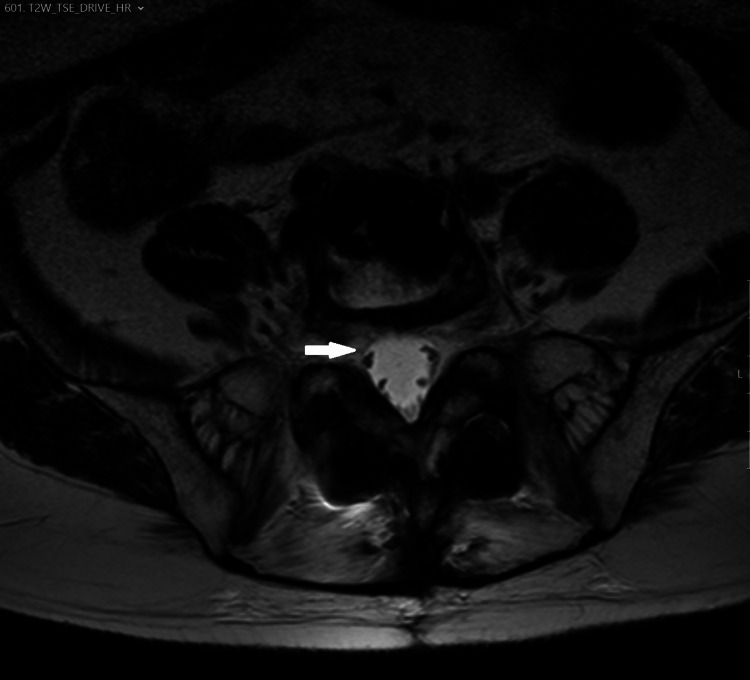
MRI after surgery, axial T2WI. Axial T2WI showed no soft tissue mass on the vertebral canal or foramina at this level. The left L5 nerve root appeared normal.
